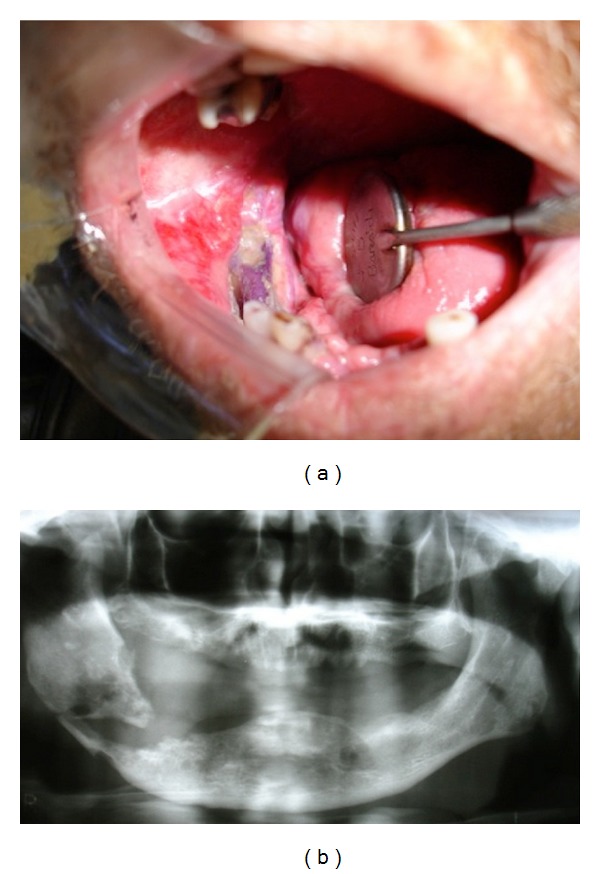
(a) Clinical aspects of osteoradionecrosis showing bone exposure of the operated area. (b) Panoramic radiograph showing pathological fracture in the mandible.
